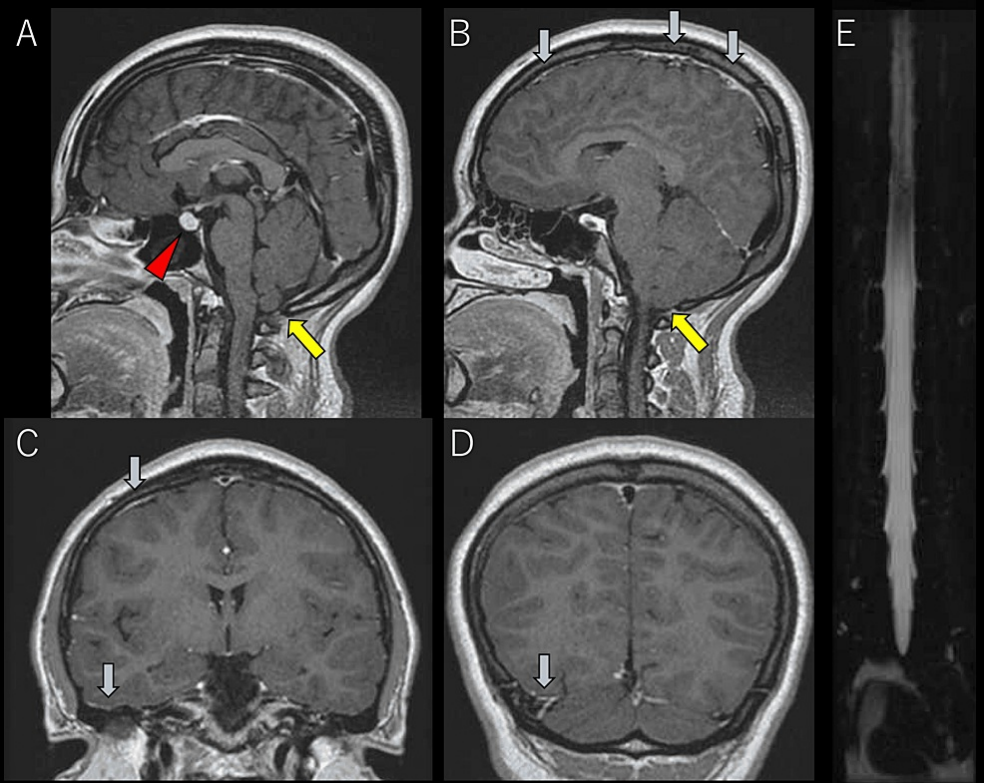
Magnetic resonance imaging The gadolinium contrast-enhanced T1-weighted magnetic resonance (MR) imaging showed partially enhanced dura matter (blue arrows in A, B, C, and D), and low-lying tonsils (yellow arrows in A and B), and pituitary enlargement (red arrowhead in A). The MR myelography could not detect findings specific to cerebrospinal fluid leakage; floating dural sac sign, incomplete floating dural sac sign, nor dinosaur tail sign (E).
